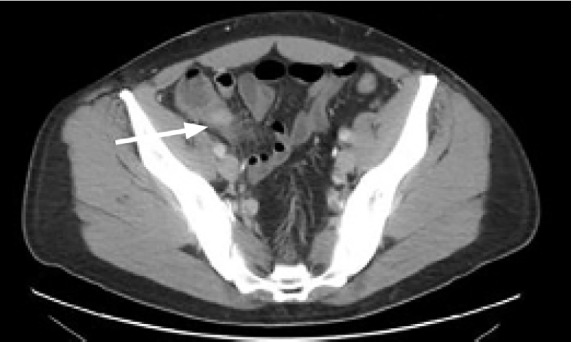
Transverse computed tomography of the abdomen and pelvis of 37-year-old male with intermittent fever and vague abdominal pain with ruptured appendicitis (arrow).
